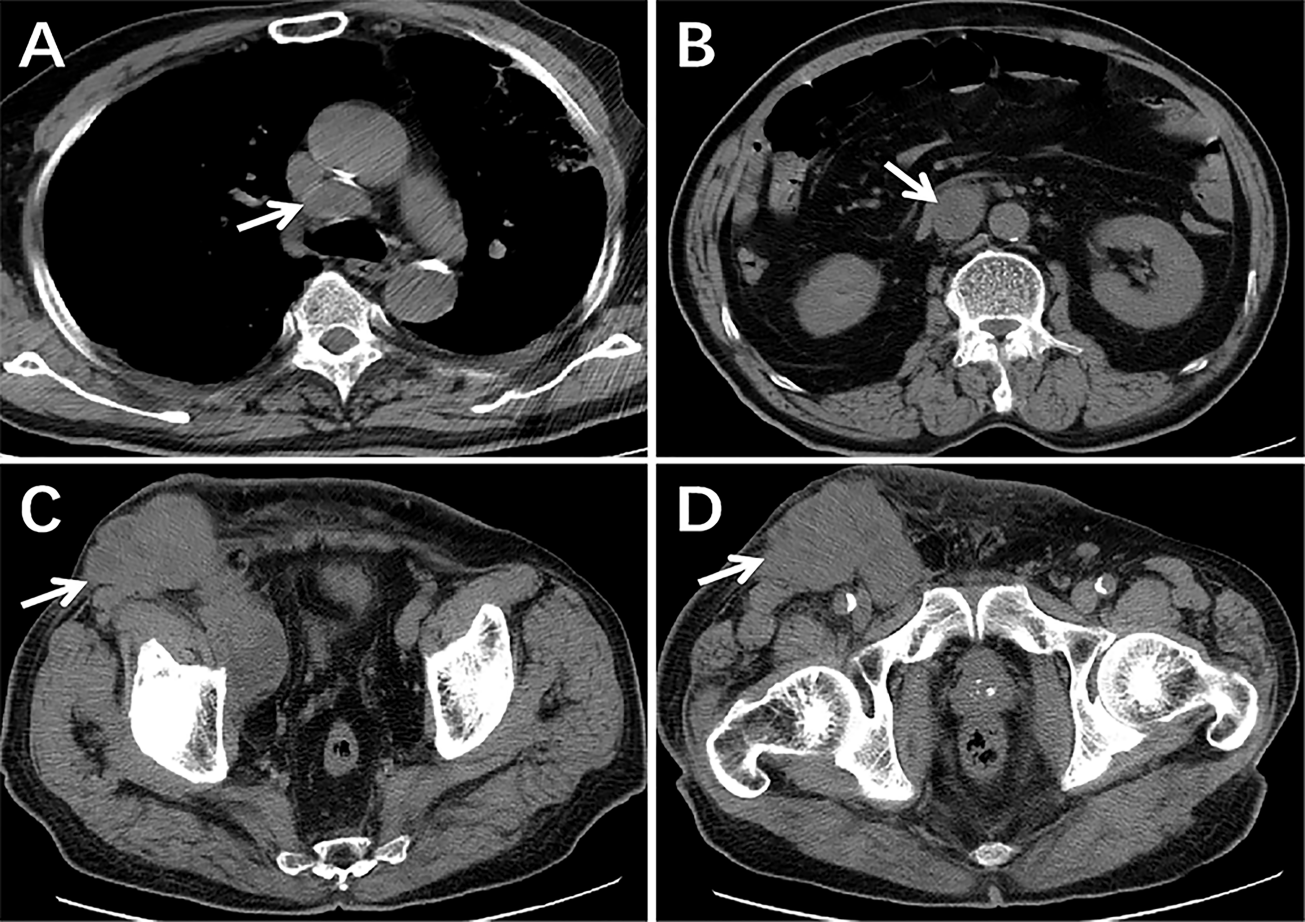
The CT scans following two cycles of treatment. There were still lesions in the mediastinum, retroperitoneum, pelvic, and inguinal regions and the size and quantity of lymph nodes had not decreased significantly from pre-treatment imaging (arrows) (A–D).
